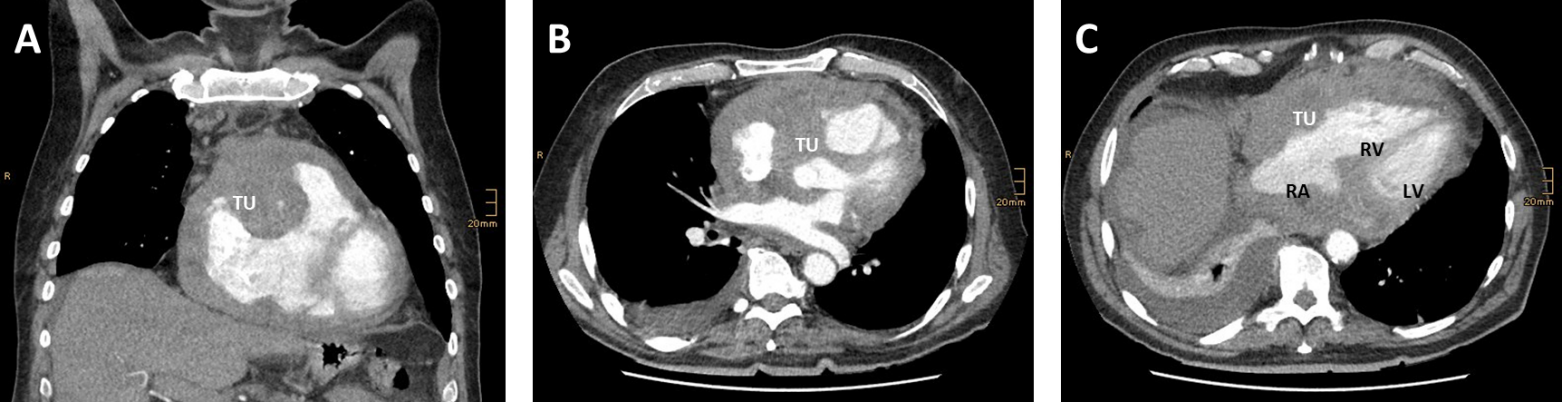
Transversal (A,B) and coronal (C) reconstructions of a computed tomography (CT)
